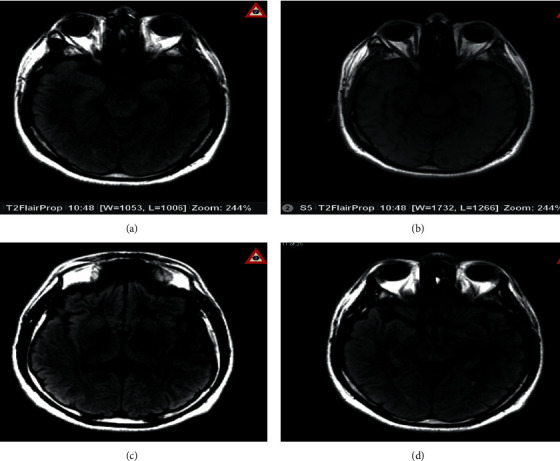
Axial magnetic resonance images (MRIs) demonstrating bright signal intensity and gyriform swelling in the region of the uncus (a) and thalami and insular cortex (b). This is best appreciated on flair images (c) and is harder to detect on T2-weighted images. The contrast study (d) does not show enhancement.
